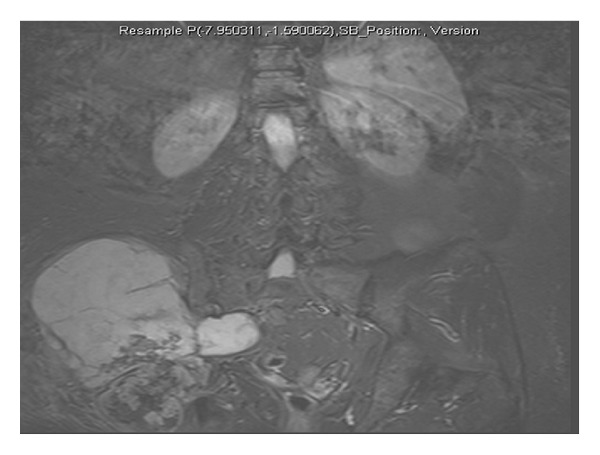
STIR MRI of case 4 preoperatively.
